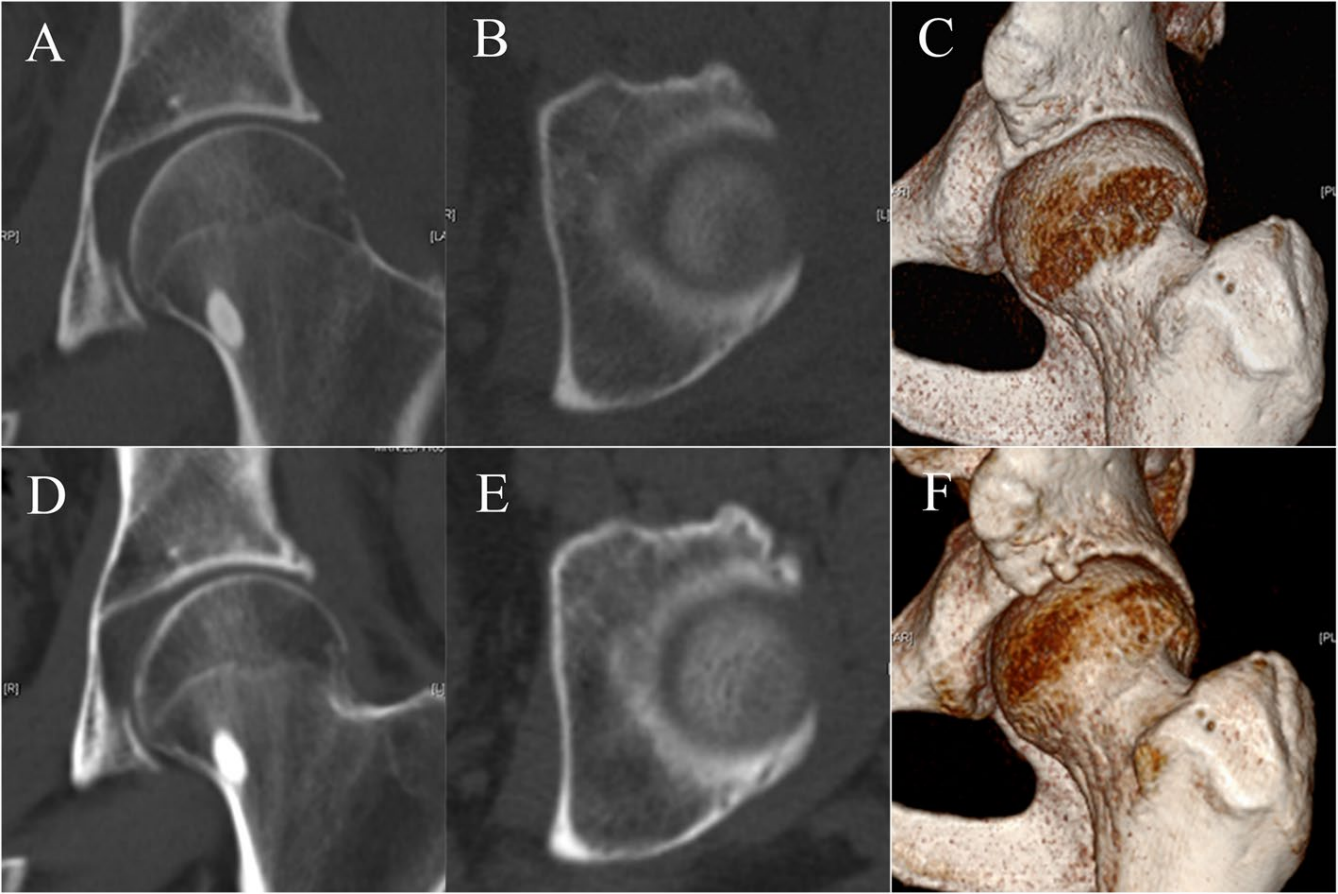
A, D. Coronal CT 1 day after surgery and at final follow-up. B, E. Axial CT 1 day after surgery and at final follow-up. C, F. 3D reconstruction CT 1 day after surgery and at final follow-up
